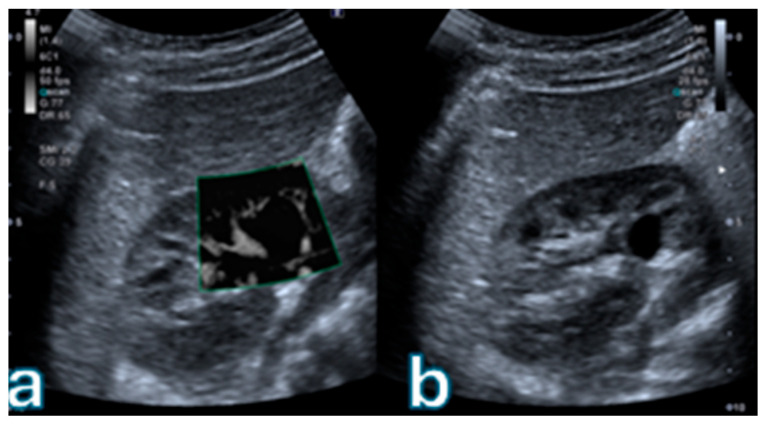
(a) Superb Microvascular Imaging (SMI) of a renal cyst demonstrates no detectable microvascular flow within the thin cyst wall, with absence of septa or mural nodules. (b) Corresponding B-mode ultrasound image shows an anechoic lesion with smooth margins and posterior acoustic enhancement, without any solid components. Overall features are consistent with a simple renal cyst (Bosniak I) according to the 2019 Bosniak classification.
